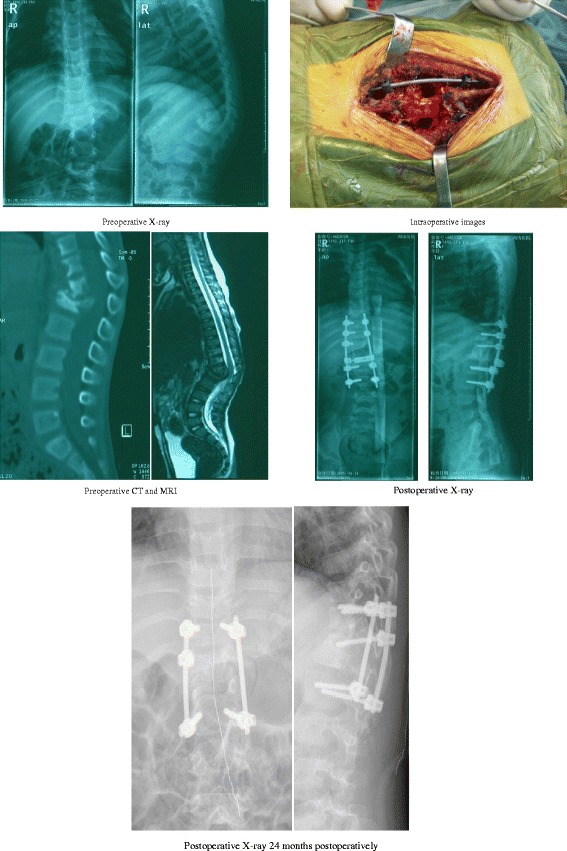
Female, 4 years, T11 and T12, L1 TB with paravertebral cold abscess formation. Preoperative X-ray AP and lateral view, kyphotic Cobb angle of 45°; preoperative two-dimensional sagittal CT, T12 and L1 vertebral severe destruction; preoperative MR, spinal cord was compressed at the T12, L1 level; Intraoperative images, anterior TB lesion debridement, titanium mesh implantation with autologous bone graft + posterior pedicle osteotomy and pedicle screw fixation; postoperative X-ray AP and lateral view, pedicle screws fixation at T10, T11, T12, L2, L3, autogenous bone grafting and fusion range limited between T11, T12 and L2 with kyphotic Cobb angle 8°; postoperative X-ray AP and lateral view 24 months postoperatively, T10, L3 pedicle screws were removed, keeping T11, T12, L2 vertebral pedicle screw fixation; successfully bone fusion range limited between T11, T12, and L2 with kyphotic Cobb angle 8°
